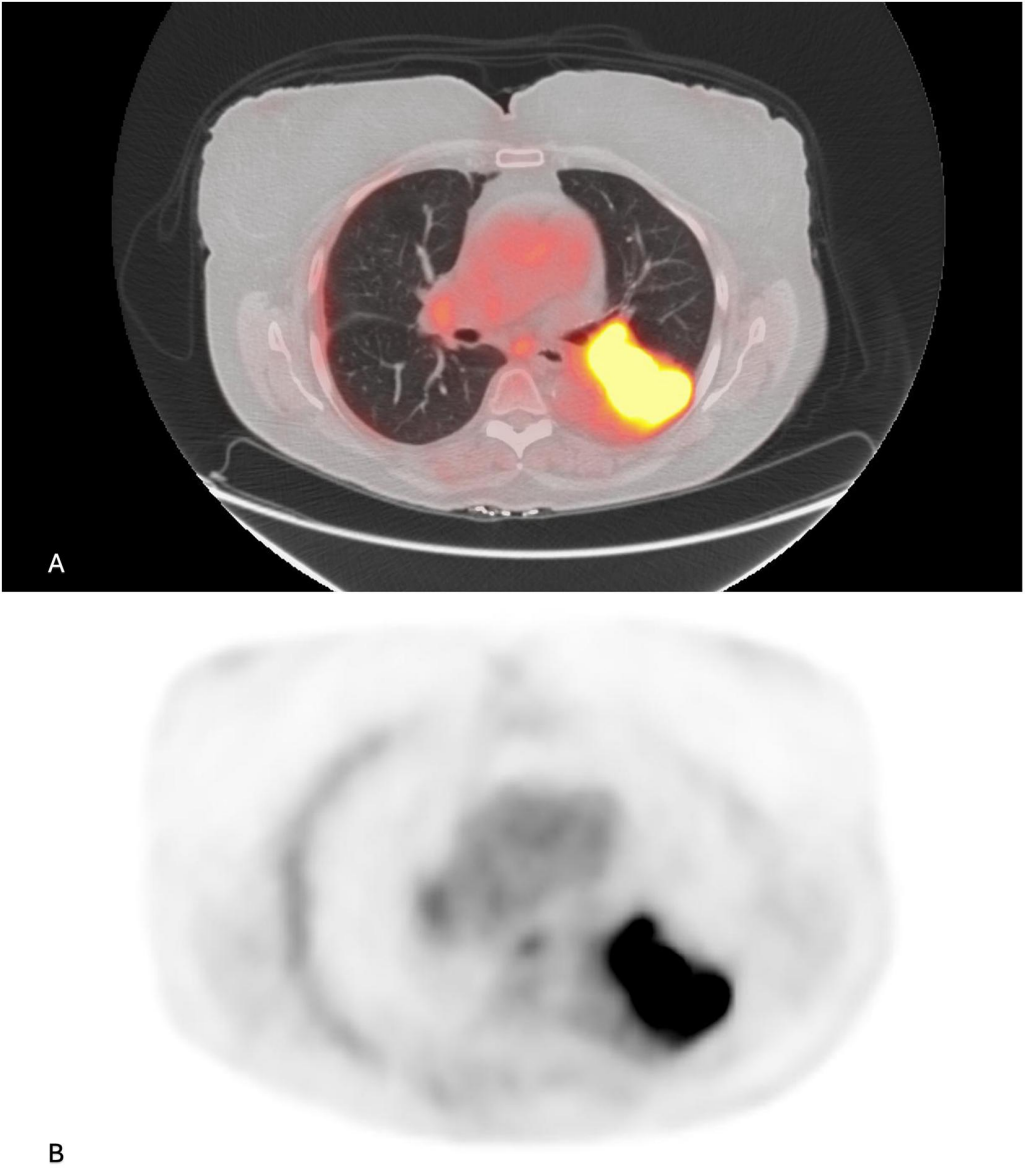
(A) Fused FDG PET/CT and (B) PET images show a lobulated, FDG-avid, left lower lobe lung mass (SUV max: 24.89).
